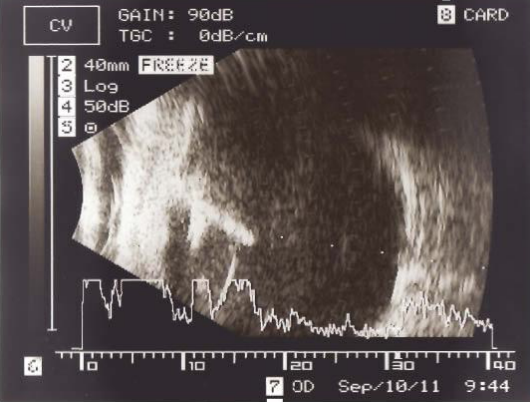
Ultrasonography showed an IOFB in the right eye
